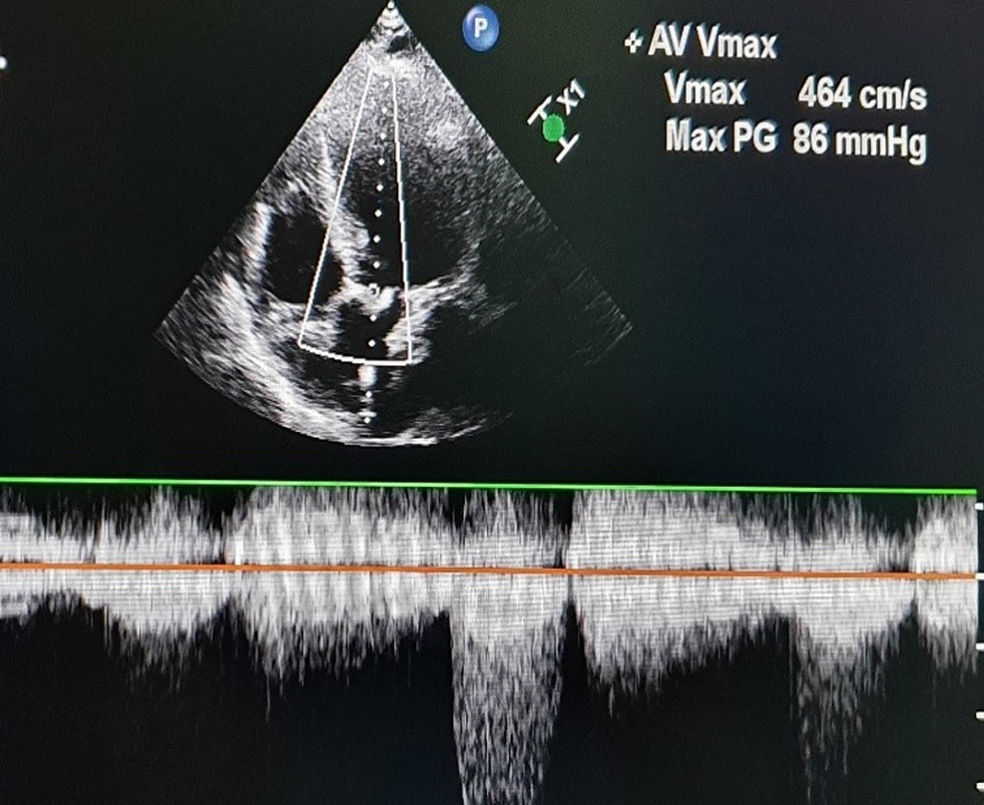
Apical five-chamber view demonstrating severe aortic stenosis with a maximum gradient of 86 mmHg.
